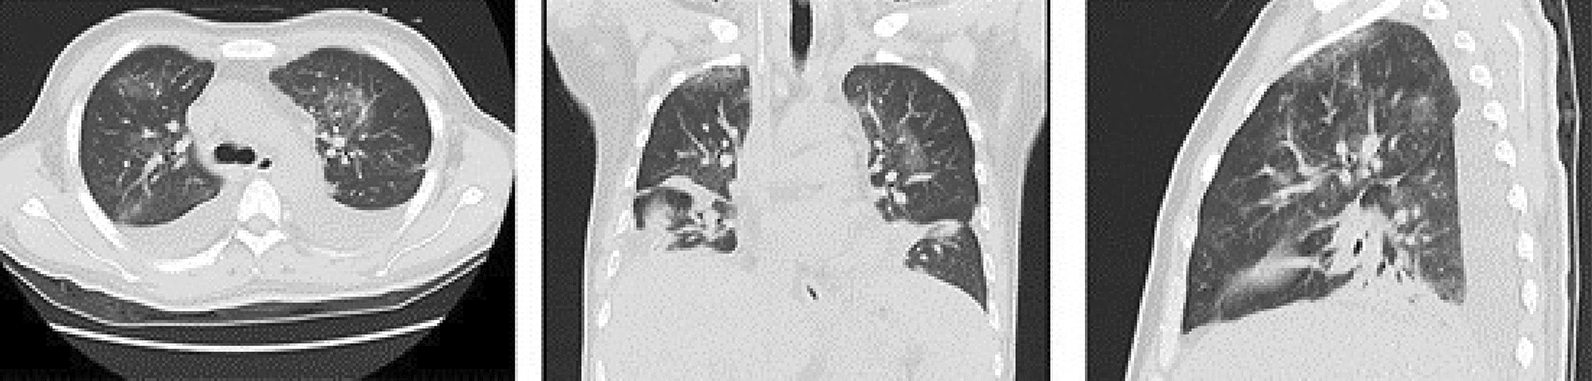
Pulmonary computed tomography showed extensive inflammatory lesions in both lungs
